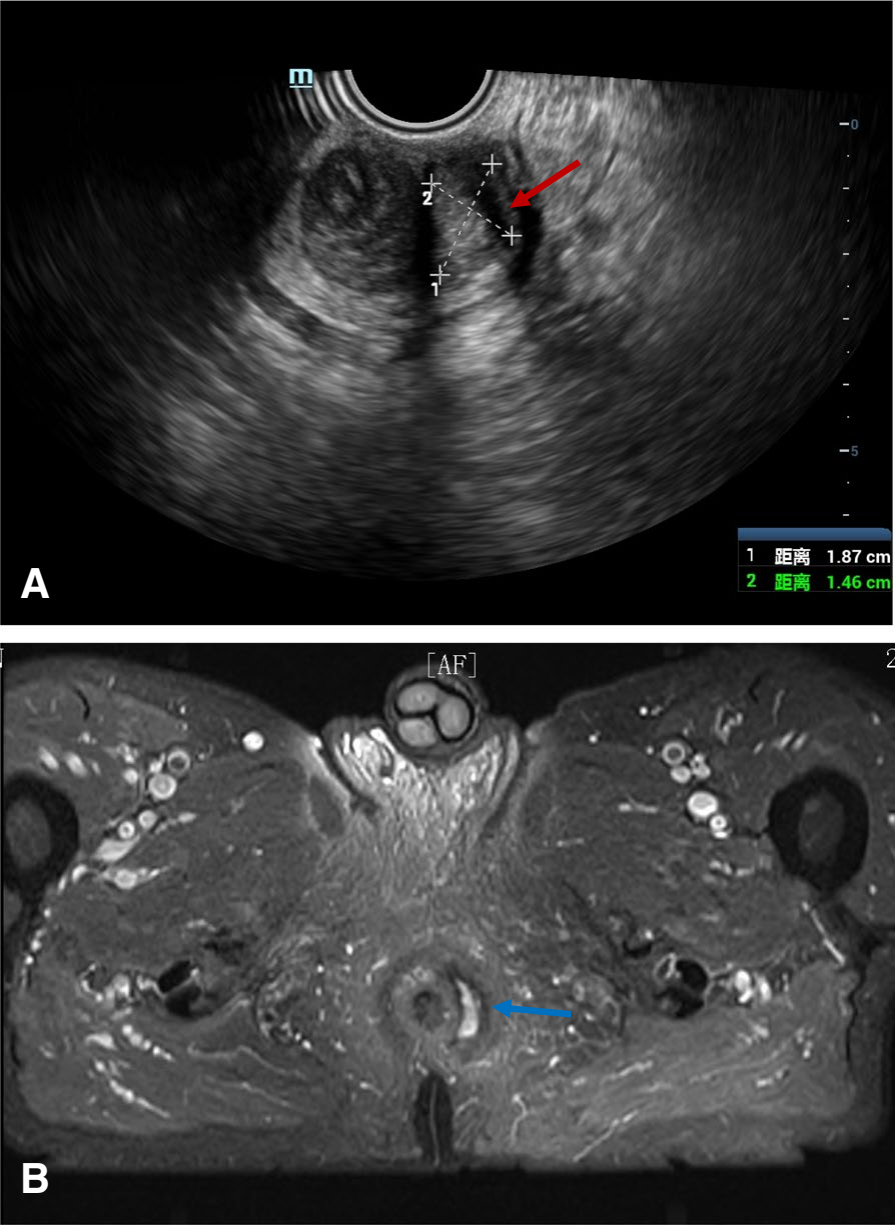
Anal fistula type I in pelvic 3D US and MRI images. A Pelvic 3D US image of a anal fistula(red arrow); (B)MRI image of a anal fistula(blue arrow)
